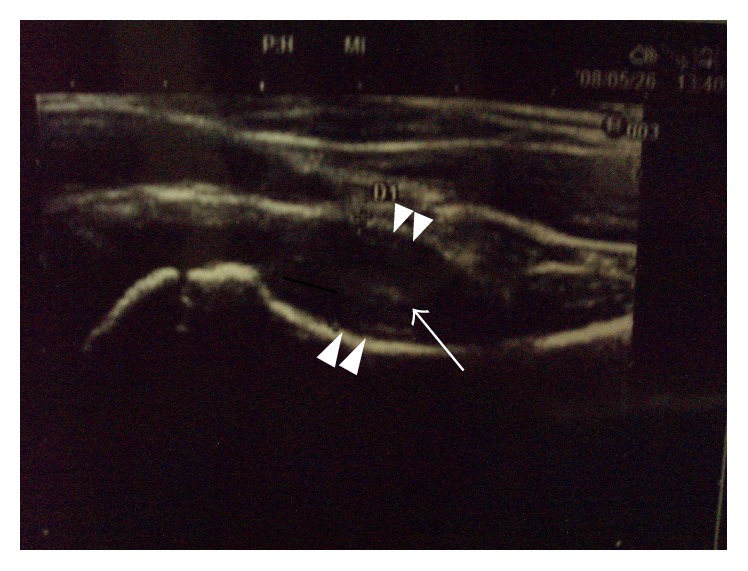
Ultrasonic joint space (distance from the anterior cortex of the femoral neck to the front of the capsule [the width of the joint space]) 2 months after the initial visit. It was 11.6 mm for the left hip (arrowheads). The intracapsular space showed echoic findings (arrow).
